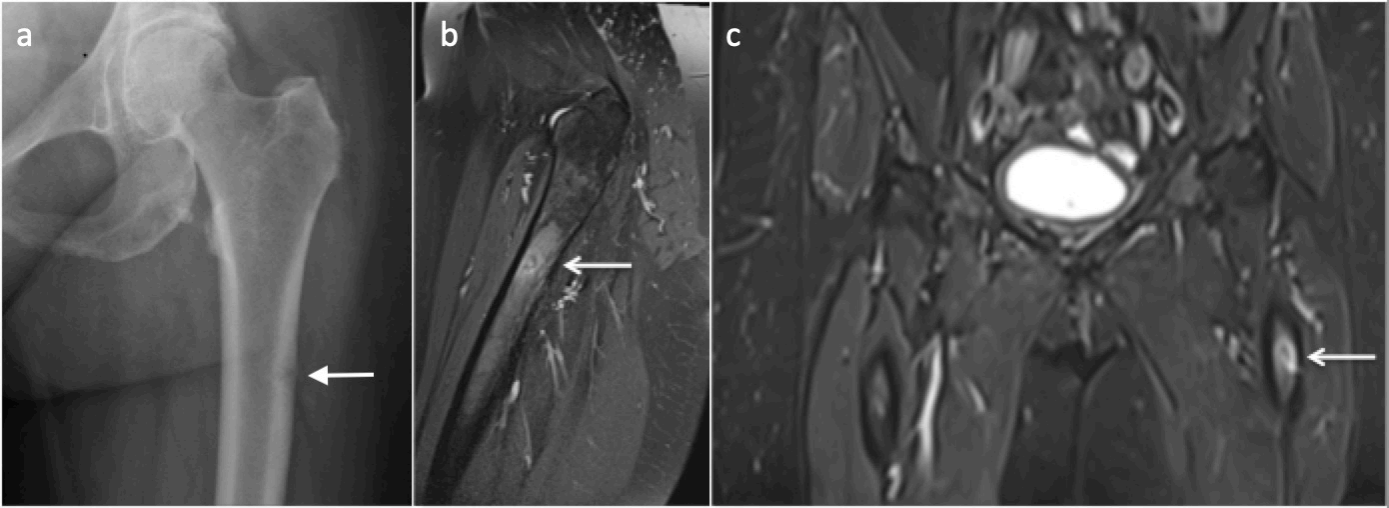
56 year-old-female with hypophosphatasia with atypical femoral fracture. Anteroposterior radiograph of the femur (a) demonstrates a lateral diaphysis lucency and MRI PDFS sequences (b, c) transverse cortical lucency (arrows) along the lateral femoral cortex. PDFS, proton density fat saturated.
